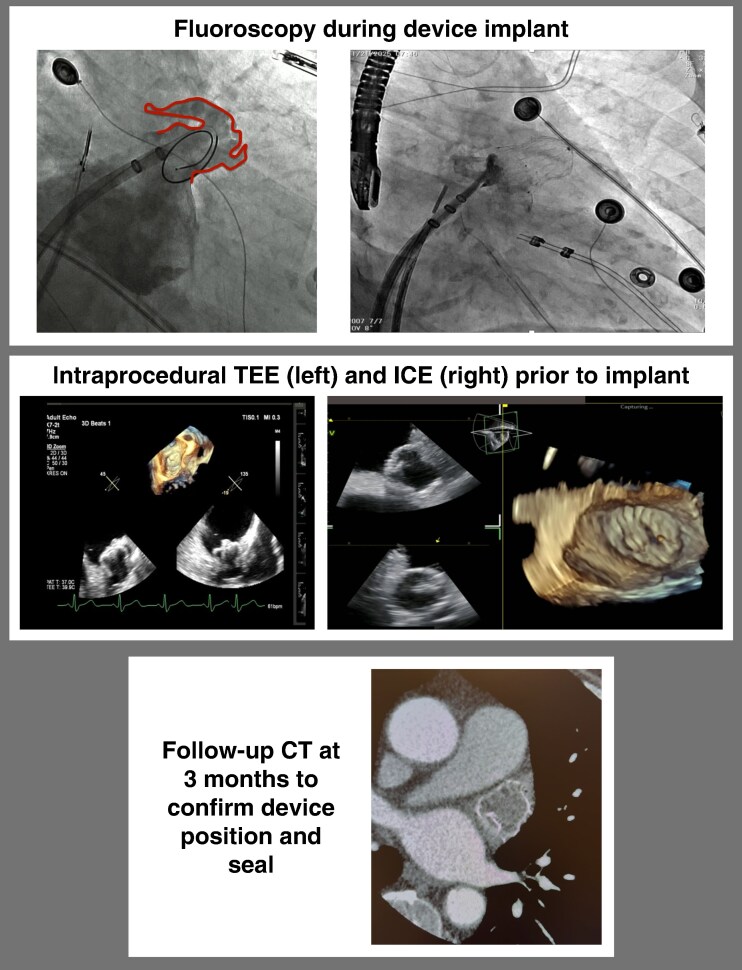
Intraprocedural and follow-up imaging for LAAC device assessment. Panel 1 shows fluoroscopic imaging during device implantation. Panel 2 shows intraprocedural TEE and ICE used to confirm device position and assess release criteria prior to implantation. Panel 3 shows follow-up CT imaging at approximately 3 months used to assess device position and peri-device seal; CT was performed in 80% of patients for leak assessment, with TEE used in the remaining 20%.
